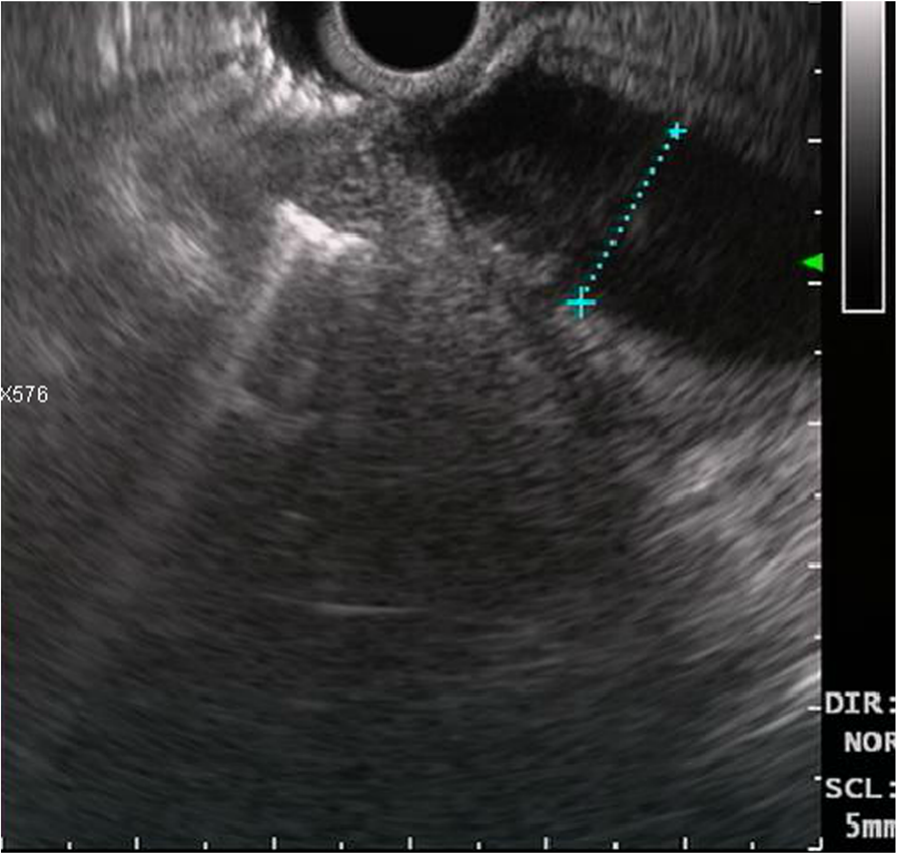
Endoscopic ultrasound showing a marked dilation of the pancreatic main duct
